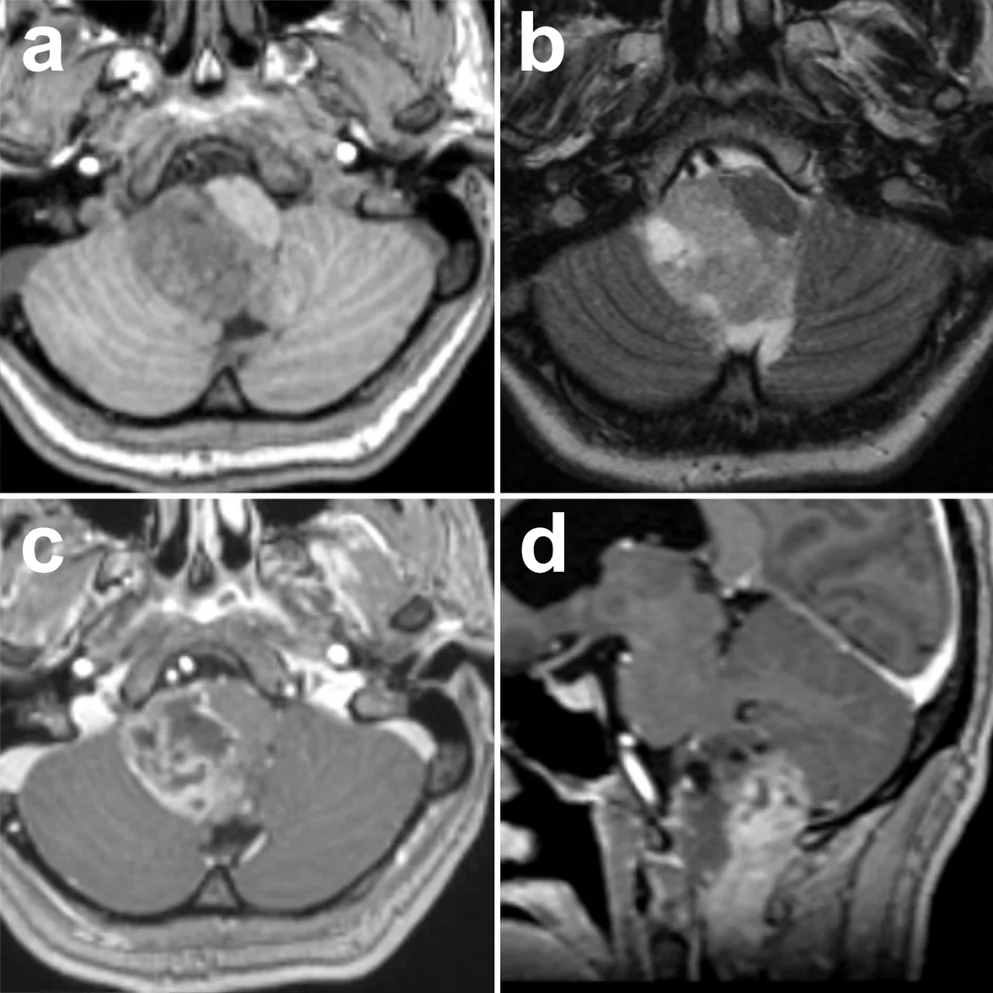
A 37-year-old woman with posterior fossa ependymoma, molecular subtype PFB, presenting with headache. Axial T1-weighted image (a), axial T2-weighted image (b), and contrast-enhanced axial and sagittal T1-weighted images (c and d, respectively). The tumor is centered in the right foramen of Luschka and caudally through the foramen magnum to the C3 level of the spinal canal. It consists of mixed solid and cystic components with heterogeneous enhancement. No apparent hemorrhage or calcification is observed. The medulla is compressed, resulting in obstructive hydrocephalus
